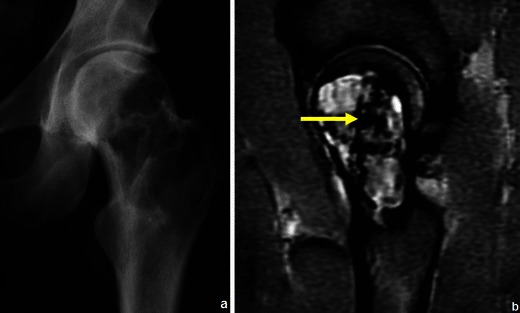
Giant cell tumour of the proximal femur in a 36-year-old patient: the coronal T2WI (b) shows a predominantly hyperintense lesion containing areas of low signal intensity; corresponding radiograph in (a)
